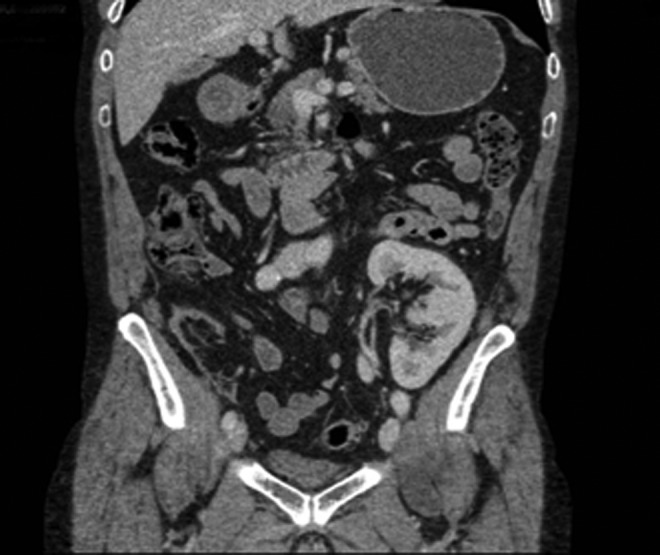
Case 2—CT urogram; coronal image showing the lateral pelvic position of the renal transplant.
